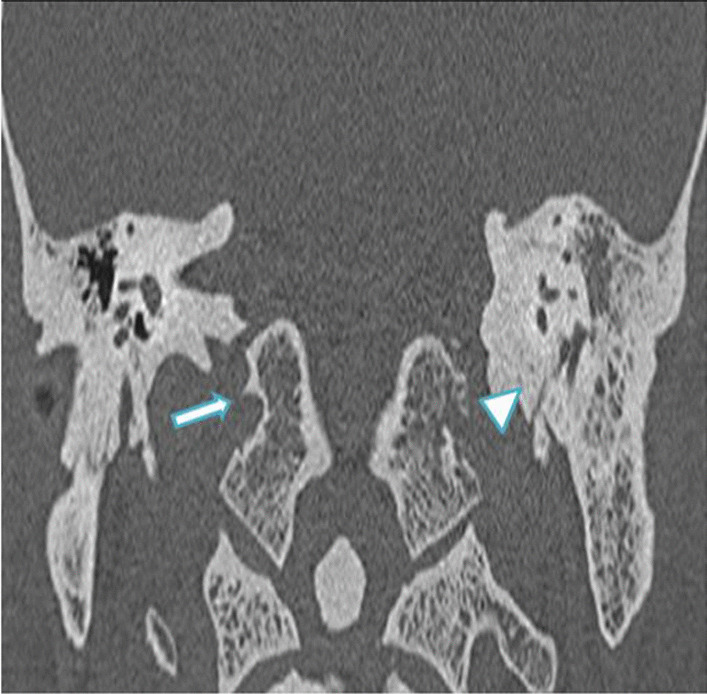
Coronal HRCT temporal bone image of a 59-year-old male patient with invasive oto-mastoiditis and resultant skull base osteomyelitis showing erosions of left hypoglossal canal and jugular tubercle (arrow head). Right hypoglossal canal and jugular tubercle is normal (arrow)
